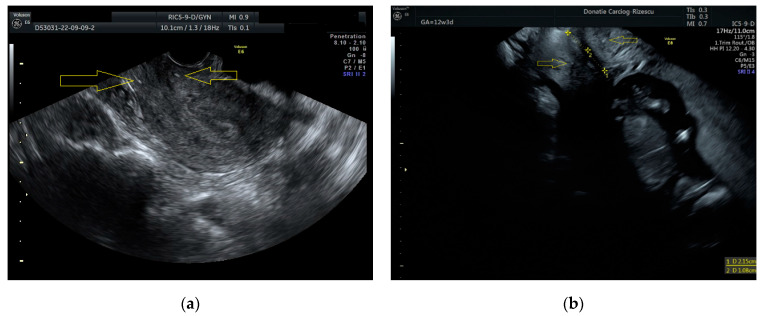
Patient no. 2. (a) Transvaginal examination of the non-pregnant uterus after RT and cerclage. The thread of cerclage is identified as two hyperechogenic spots (arrows). (b) Transvaginal ultrasound in the same patient (while 12 weeks pregnant) revealed a total cervical length of 21.5 mm, with an internal distance of 10.7 mm and external distance of 10.8 mm.
